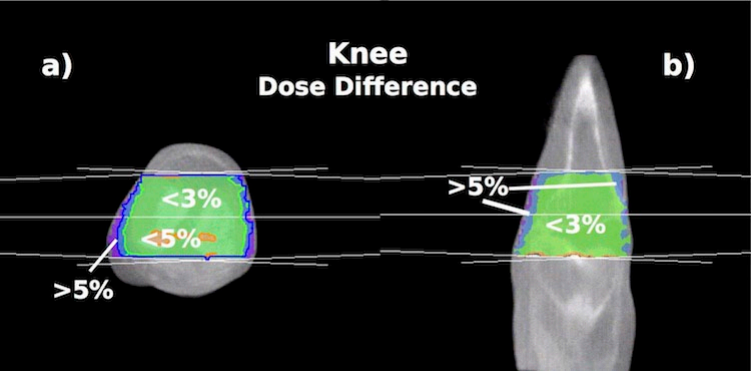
Dose difference for a knee plan: (a) transverse and (b) coronal views of a knee showing the dose difference relative to a standard CT planned on a 4.5 MU exposure rFOV MV CBCT. Opposed lateral beams with equal weighting were used in this plan.
